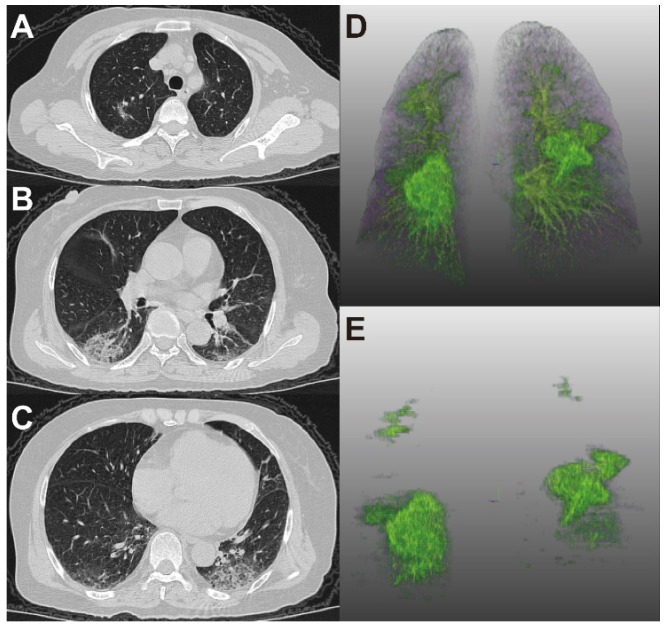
66-year-old woman with coronavirus disease 2019. A-C. Non-contrast CT was performed on day of admission. D. Three-dimensional volume-rendered reconstruction shows the distribution of the opacities. E. Pulmonary opacities segmented by AI system. The patient showed fever and cough at admission. Patient did not reach clinical endpoint during hospitalization. Lung CT images showed that consolidation and ground-glass opacities mainly distributed in the lower lungs.
